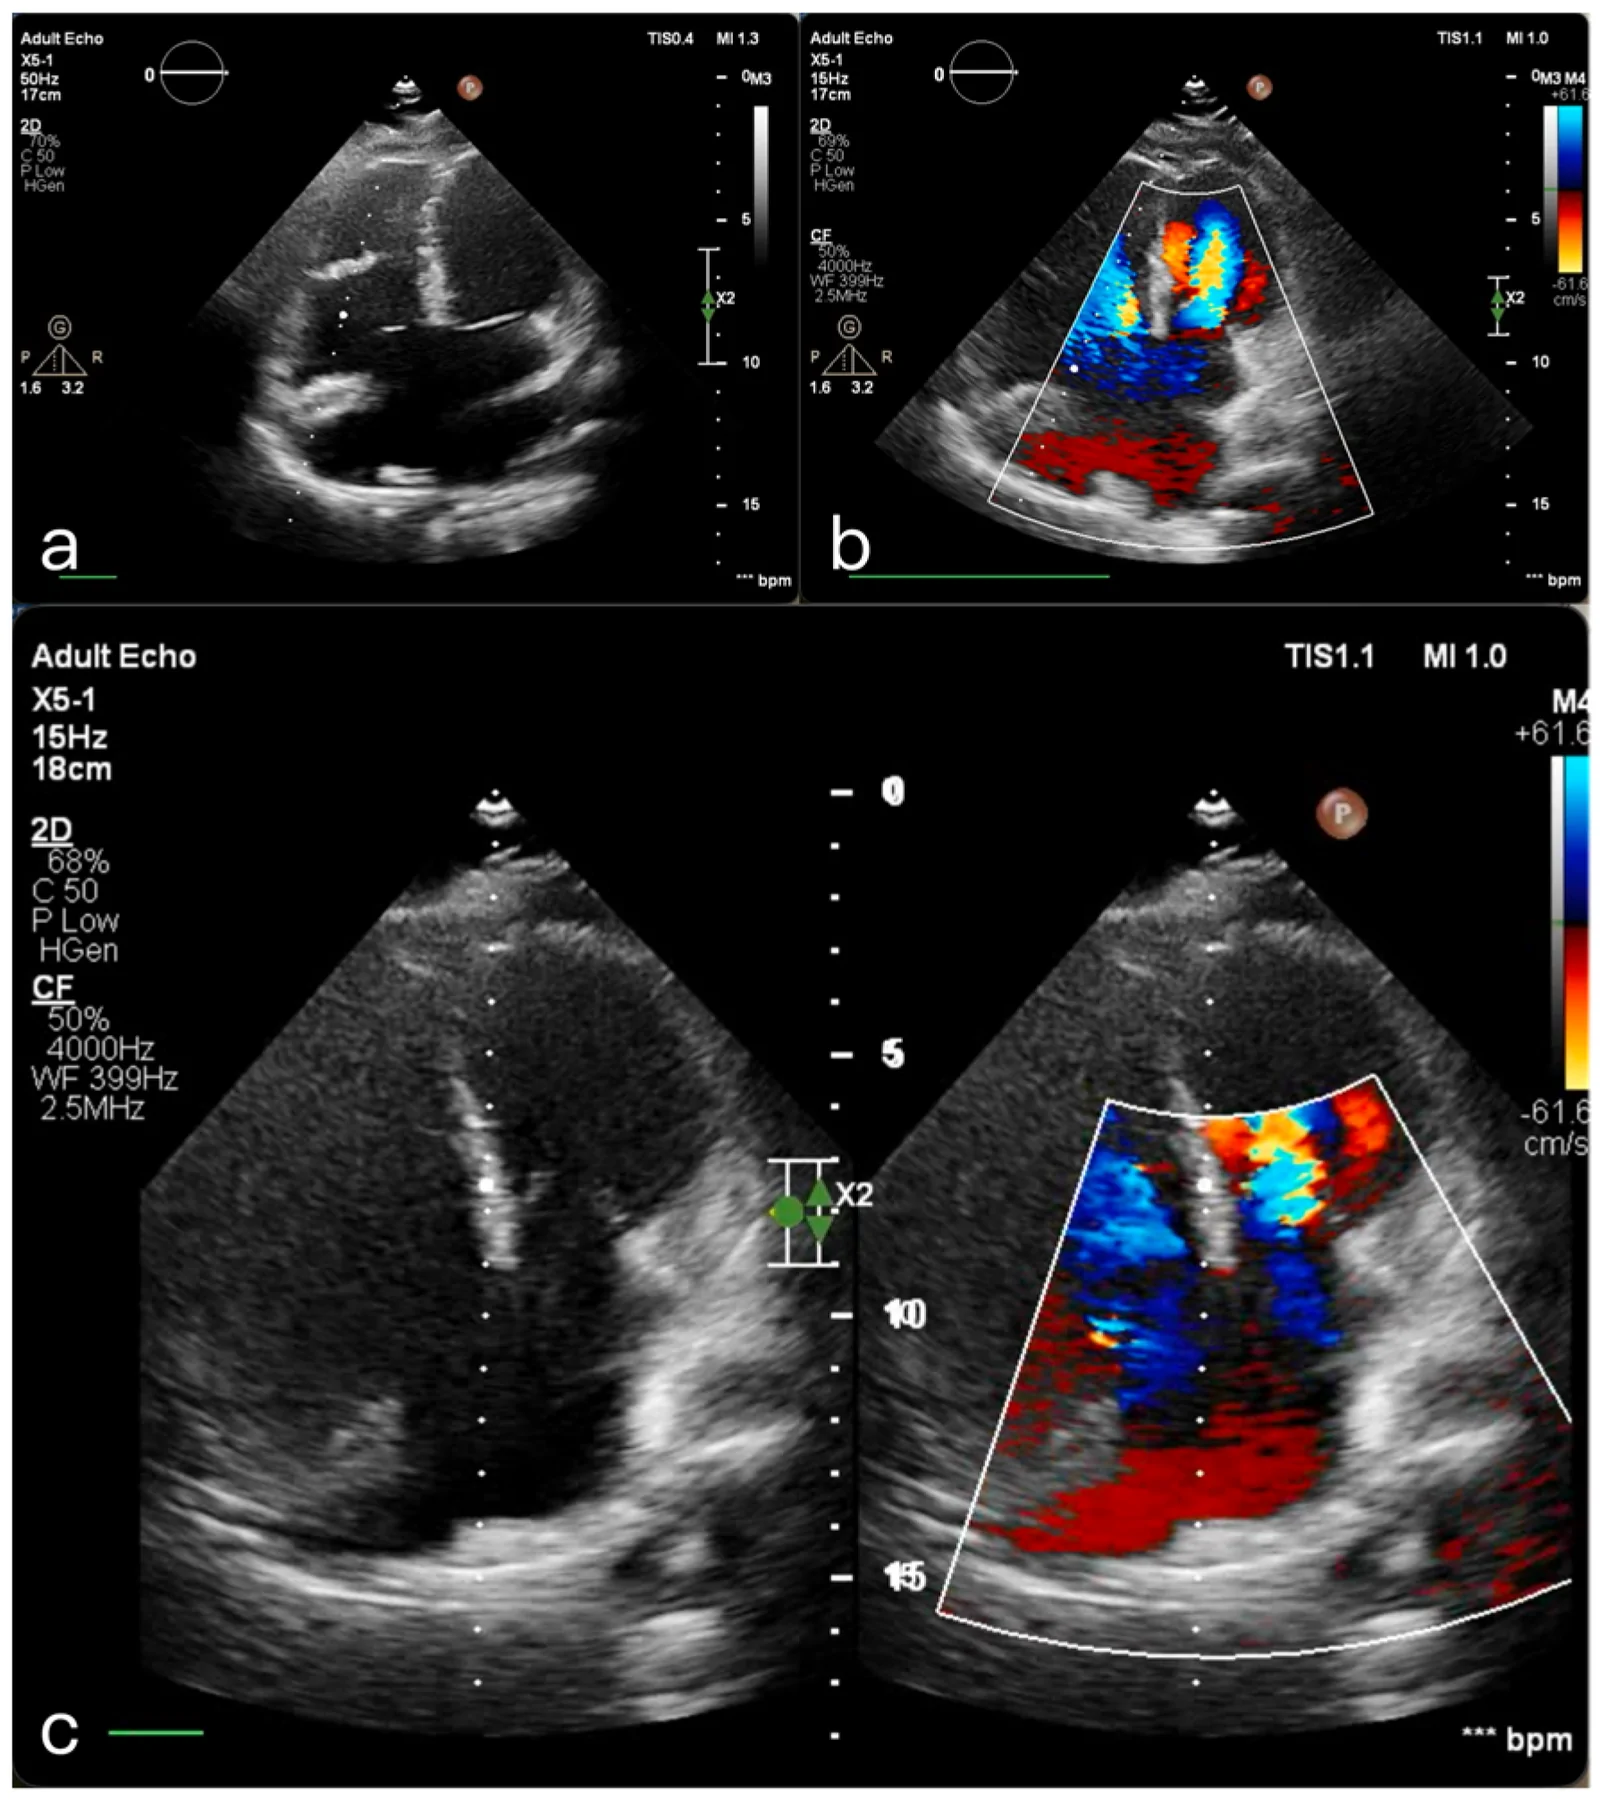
Preoperative transthoracic echocardiography (TTE) (apical four-chamber view) reveals a single atrium. (a) Two-dimensional imaging shows complete absence of the interatrial septum, with a common atrial chamber. (b) Color Doppler demonstrates bidirectional shunting across the common atrium. (c) Side-by-side comparison of two-dimensional and color Doppler imaging at the same plane highlights the anatomical defect and its corresponding hemodynamic disturbance in the common atrial chamber.
